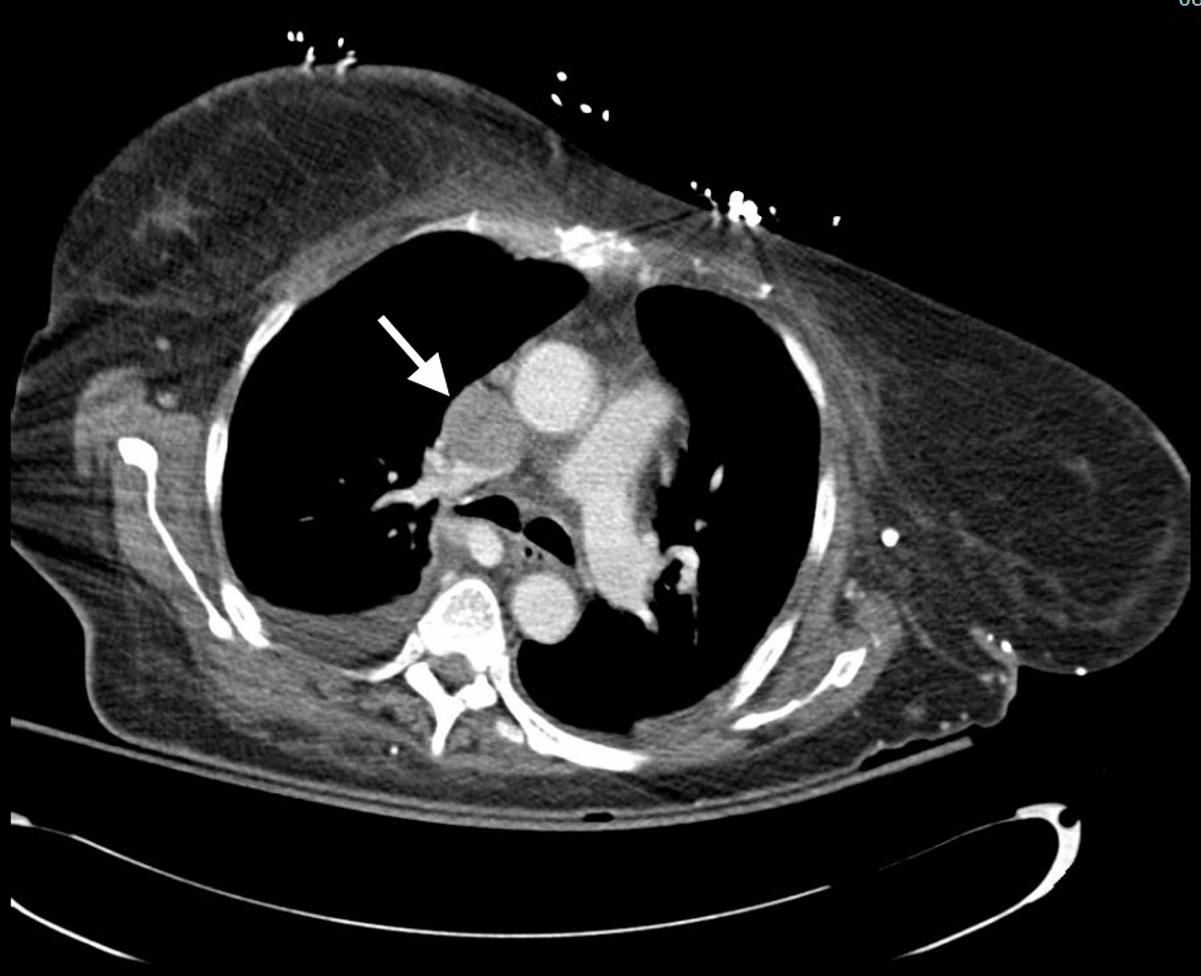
CT of chest showing a completely occlusive thrombus of the superior vena cava
